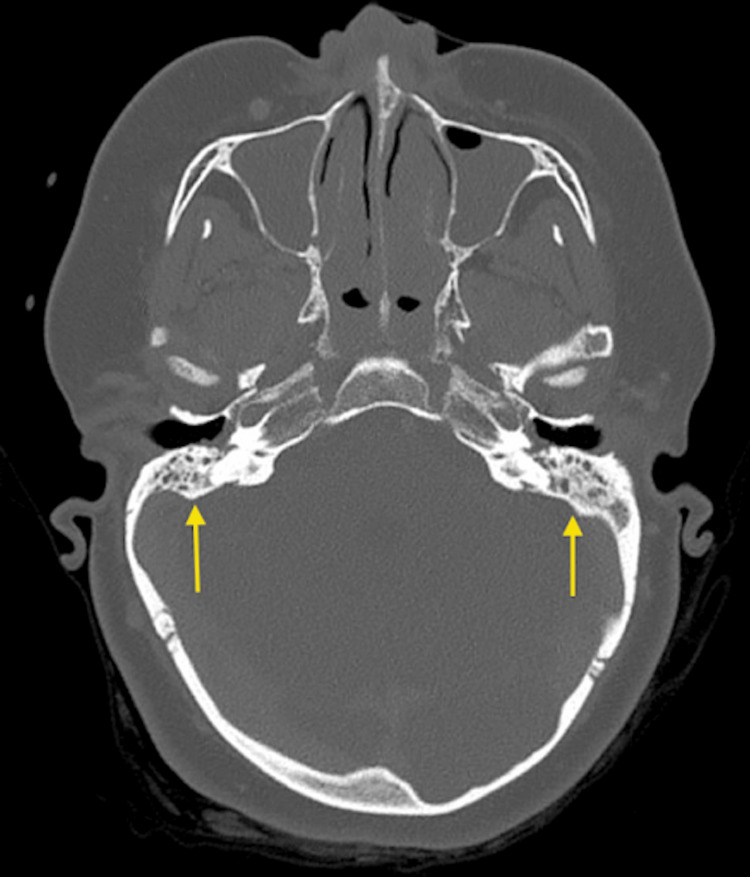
High-resolution CT Temporal Bones (axial reformat) demonstrates bilateral otomastoiditis with near-complete opacification of the mastoid air cells and middle ear cavities bilaterally (arrows). Hypoplastic and poorly pneumatised mastoid air cells were noted bilaterally, which may represent a congenital anatomical variant or changes secondary to previous otitis.
